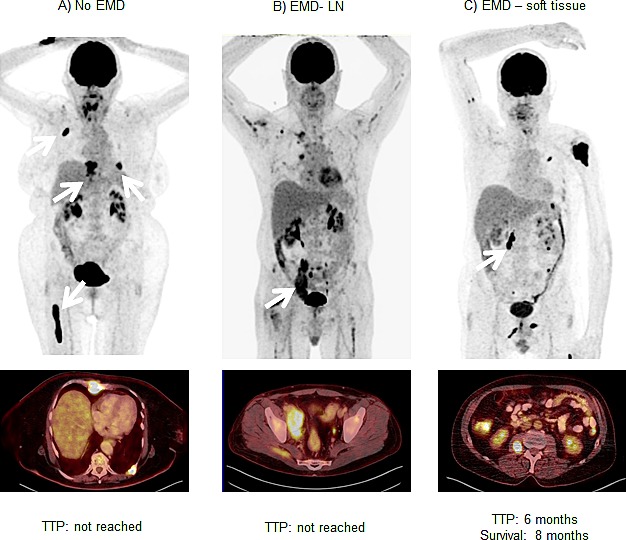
Negative prognostic value of extramedullary soft tissue lesions Display of three patients without (A) and with (B; C) extramedullary myeloma manifestations. Whereas patient A had exclusively intramedullary lesions, patients B and C presented with intramedullary and extramedullary disease. Patient B had mainly iliac lymph node involvement (arrow), patient C suffered from muscle manifestations (arrow). All patients received chemotherapy with novel agents after ^18^F-FDG-PET/CT; follow-up was 8 months in all. Whereas patients A and B have not progressed yet, patient C died 8 months after the PET scan. EMD = extramedullary disease, LN = lymph node, TTP = time to progression
